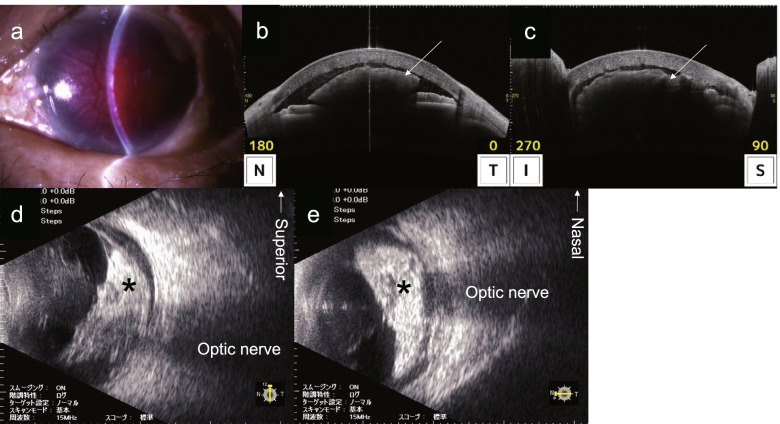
Massive intraocular hemorrhage at the initial visit to our department. Slit-lamp examination (a) and anterior segment optical coherence tomography (b, c) revealing corneal edema and massive hyphema (arrow) in the anterior chamber. A hyperechoic image indicative of vitreous hemorrhage is observed on ocular ultrasound examination (d, e). No evident findings of retinal detachment and choroidal detachment are observed
